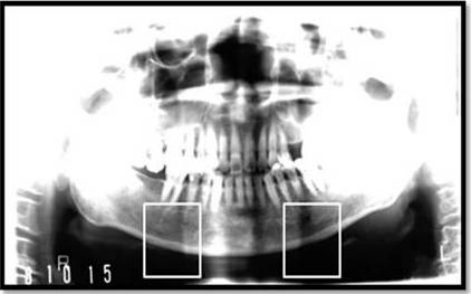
Digitized dental panoramic radiographs showing two boxes corresponding to the region of interest between the mental foramen and angle of the mandible on the right and left sides of the mandible.
